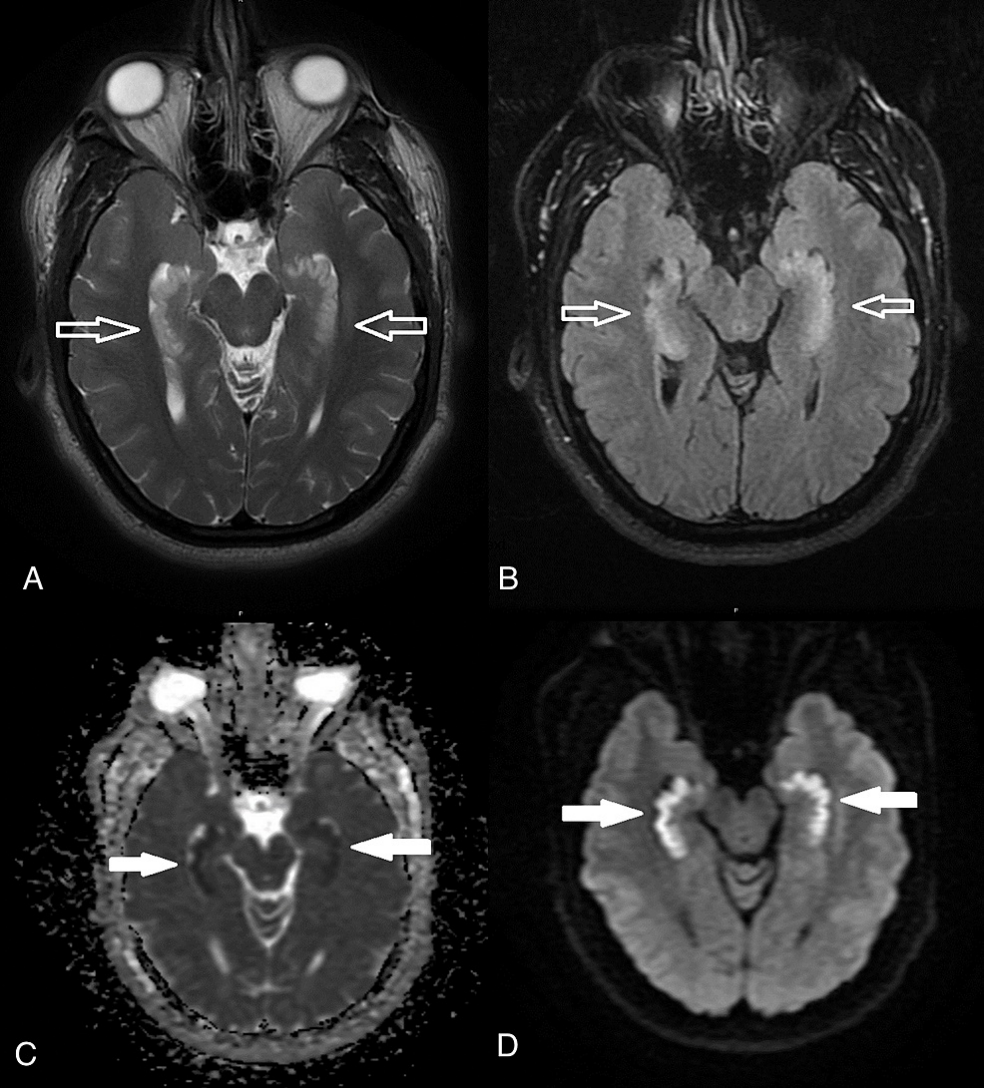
Axial views; T2-weighted (A), and FLAIR (B) demonstrate hyperintensity in the bilateral hippocampi region (white hollow arrows). On ADC (C), there is decreased signal intensity in the same hippocampi region (white solid arrows) along with corresponding increased signal intensity in DWI (D) which are suggestive of a diffusion restriction. ADC: Apparent diffusion coefficient; DWI: Diffusion-weighted imaging.
